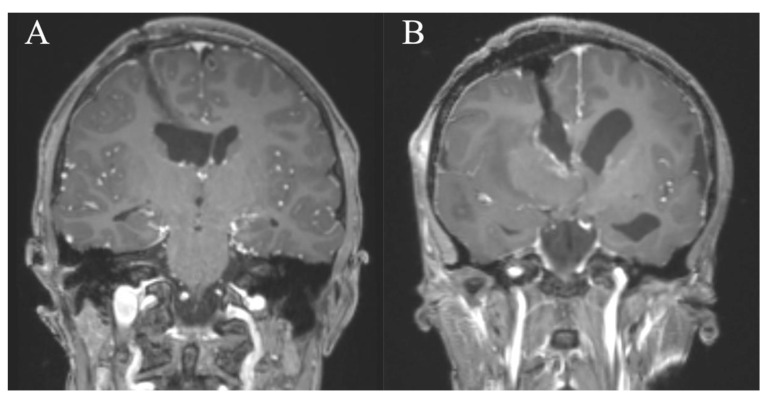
Cases 8 and 7. Coronal MR images, T1-weighted sequences with contrast medium immediately postoperatively (A,B) after resection of an intraventricular lesion through a frontal right approach in a pure endoscopic (A) and endoscopic-assisted fashion (B). A PE approach tends to result in less tissue damage to the cortex and parenchyma due to the small diameter peel-away sheath (A) compared to the endoscopic-assisted approach using the Vycor speculum (B).
